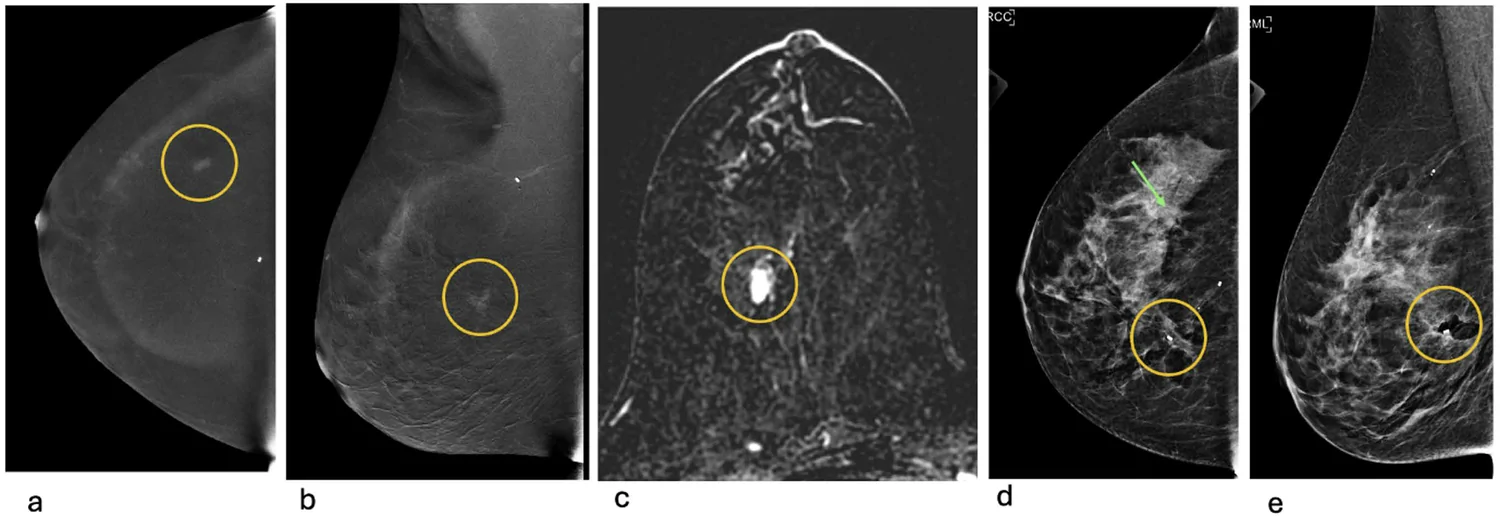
Moderate-conspicuity invasive carcinoma (not otherwise specified). A 58-year-old woman with a family history of breast cancer underwent routine screening CEM. On CEM, a moderate-conspicuity focal non-mass enhancement is seen on recombined images, on the craniocaudal (a) and mediolateral oblique (b) projections, without a correlate on the low-energy images (not pictured). Subsequent contrast-enhanced MRI (c axial post-contrast subtraction sequence) demonstrated a corresponding enhancing mass. MRI-guided biopsy was performed, yielding invasive carcinoma (not otherwise specified). Post-procedure full-field digital mammogram (d craniocaudal projection and e lateral projection) demonstrated the biopsy marker in the location of the originally identified enhancing mass
